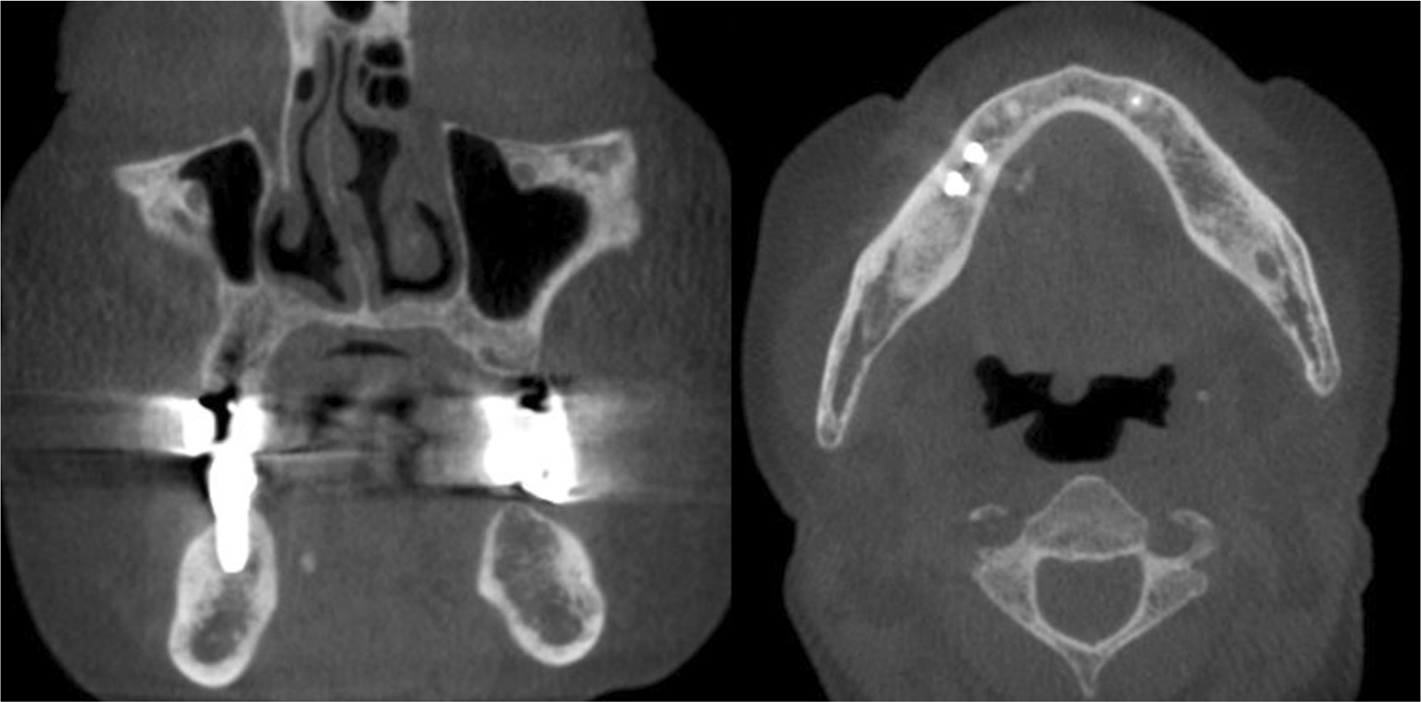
Salivary stones measuring 2 × 10 mm are detected in the right submandibular gland with cone-beam CT
